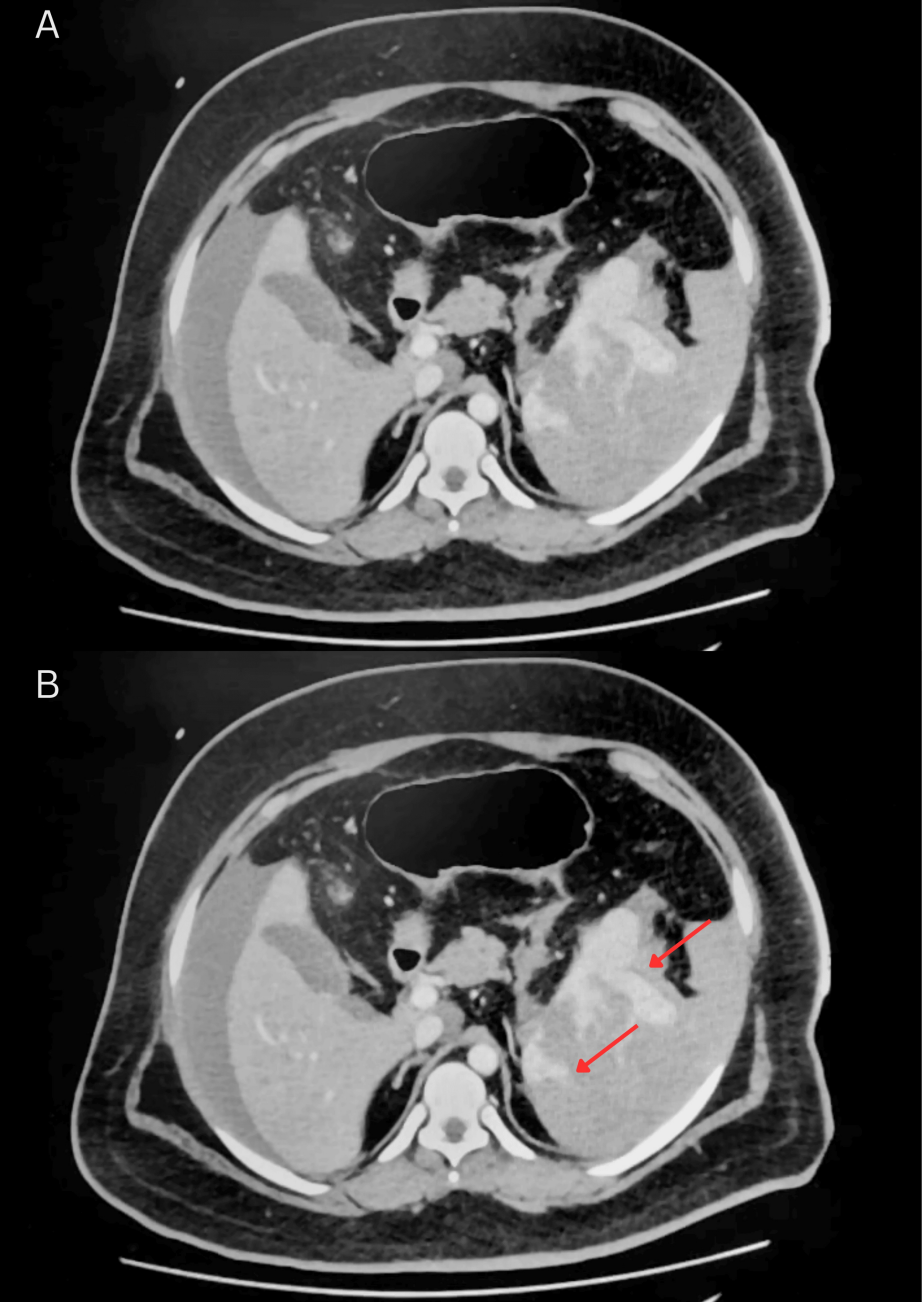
Contrast-enhanced axial venous-phase CT of abdomen demonstrating splenic rupture Pre-operative axial venous-phase contrast-enhanced CT suggestive of splenic parenchyma with focal areas of contrast extravasation (red arrows). (A) shows the unannotated scan and (B) shows the annotated scan.
